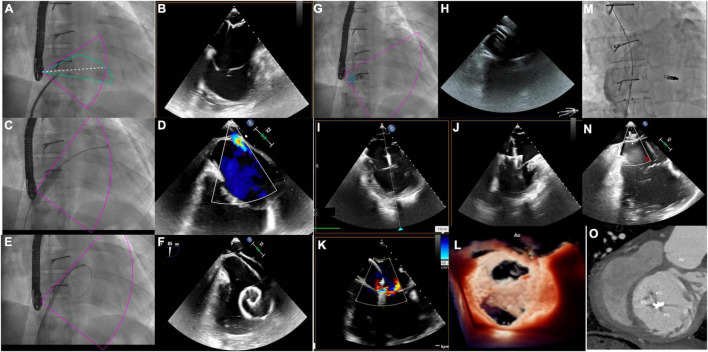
MitraClip implantation for systemic tricuspid valve regurgitation. (A,B) Catheter and needle are positioned at the level of the anterior part of the intra-atrial inferior vena cava channel under transesophageal echocardiography (TEE) guidance. The 80° TEE plane shows needle tenting and guarantees a distance of at least 40 mm between the tenting and the systemic atrio-ventricular valve (SAVV) and the alignment with the SAVV. (C) The balloon is inflated to enlarge the transbaffle access. (D) TEE shows the right to left shunt on color doppler after balloon inflation. (E,F) The guidewire is positioned in the systemic atrium to advance the MitraClip sheath. (G,H) MitraClip sheath is positioned on the systemic atrium at 40 mm or more from the SAVV. (I,J) The MitraClip system is advanced through the sheath and positioned on top of the jet origin, with the clip fully opened. Perpendicular orientation of the clip relative to the anteroseptal commissure is guided by the Xplane 3DTEE in the mid-esophagus position. (K) XTR MitraClip fully closed at the level of the anteroseptal commissure creates a double-orifice SAVV with two mild residual jets on color doppler. (L) Double-orifice SAVV on 3D Zoom true view mode after XTR clip insertion. (M) Inferior vena cava channel angiogram after placement of an 8 mm atrial septal defect (ASD) device to close the transbaffle access showing an absence of obstruction. (N) The 80° TEE view showing no residual shunt at the level of the ASD device. (O) Post- MitraClip computed tomography showing the position of the clip at the level of the anteroseptal commissure.
